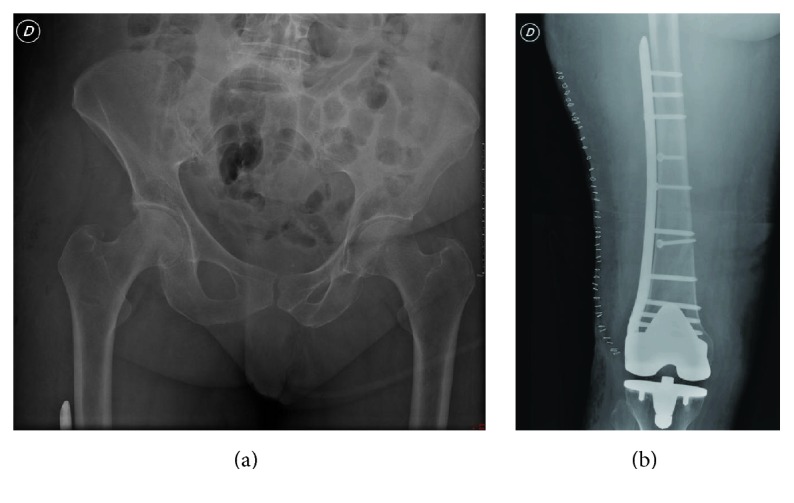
Postoperative X-ray examination.
